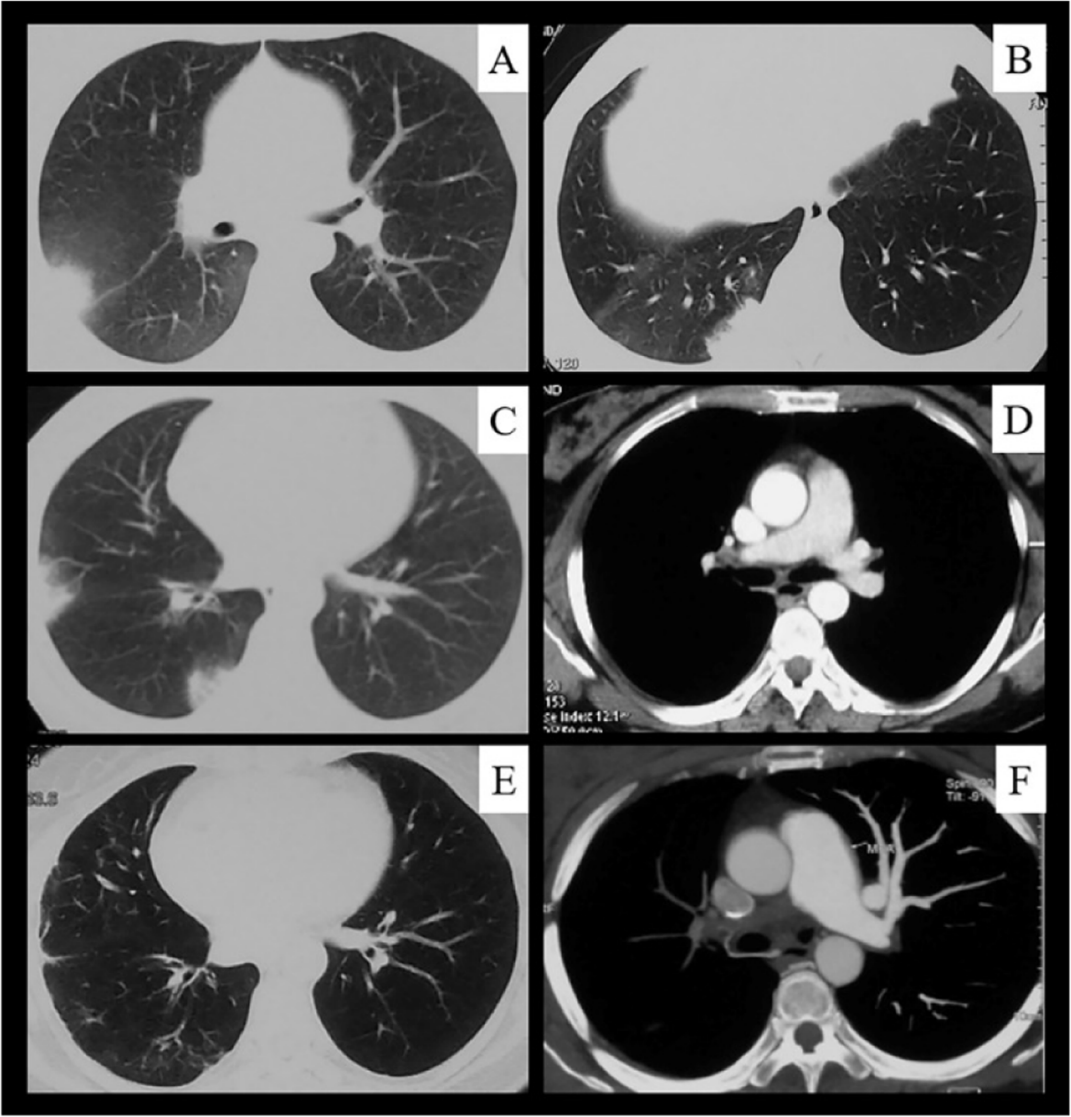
Serial computed tomography (CT) images in a 40-year-old woman with Takayasu’s arteritis. The patient was admitted to our hospital because of shortness of breath. She had developed recurrent chest and back pain and hemoptysis 4 years previously. a–c, CT images show recurrent subpleural wedge-shaped opacities during the initial 6 months after disease onset. d CT pulmonary angiography (CTPA) image obtained at the same time as in (c) showed right pulmonary artery stenosis. e, four years later, a CT image shows peripheral scarring from previous infarcts. f, CTPA image obtained at the same time showed right pulmonary artery occlusion
